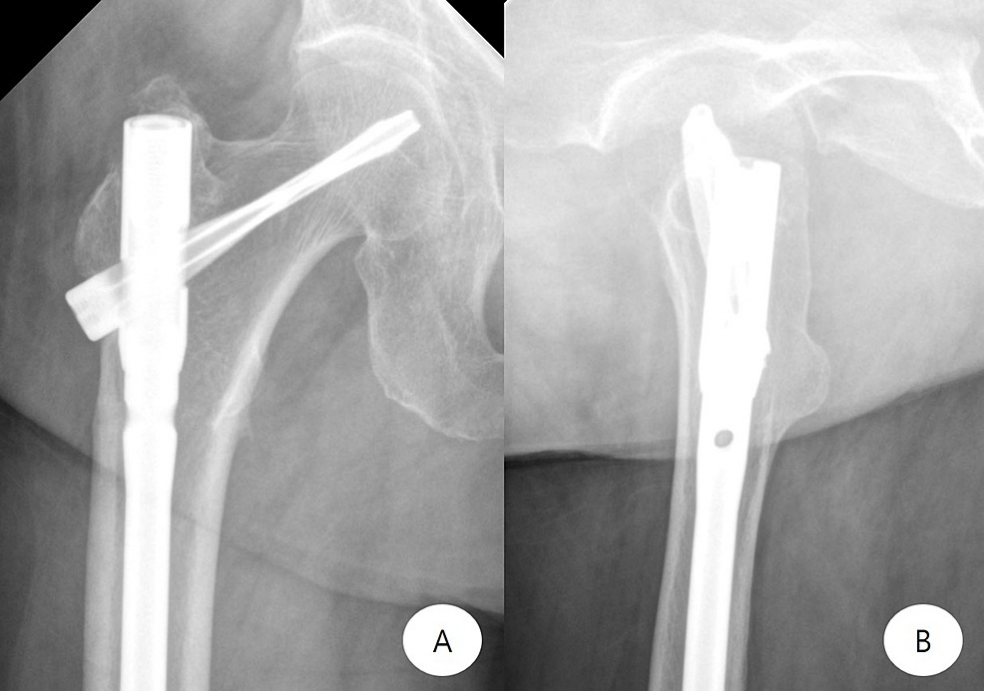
Follow-up imaging - case 1 Thirteen-month follow-up anteroposterior (A) and lateral (B) radiographs show complete healing of the previous incomplete fracture at the subtrochanteric region, incomplete fracture line, and anterior cortical thickening at the lower border of the lesser trochanter
